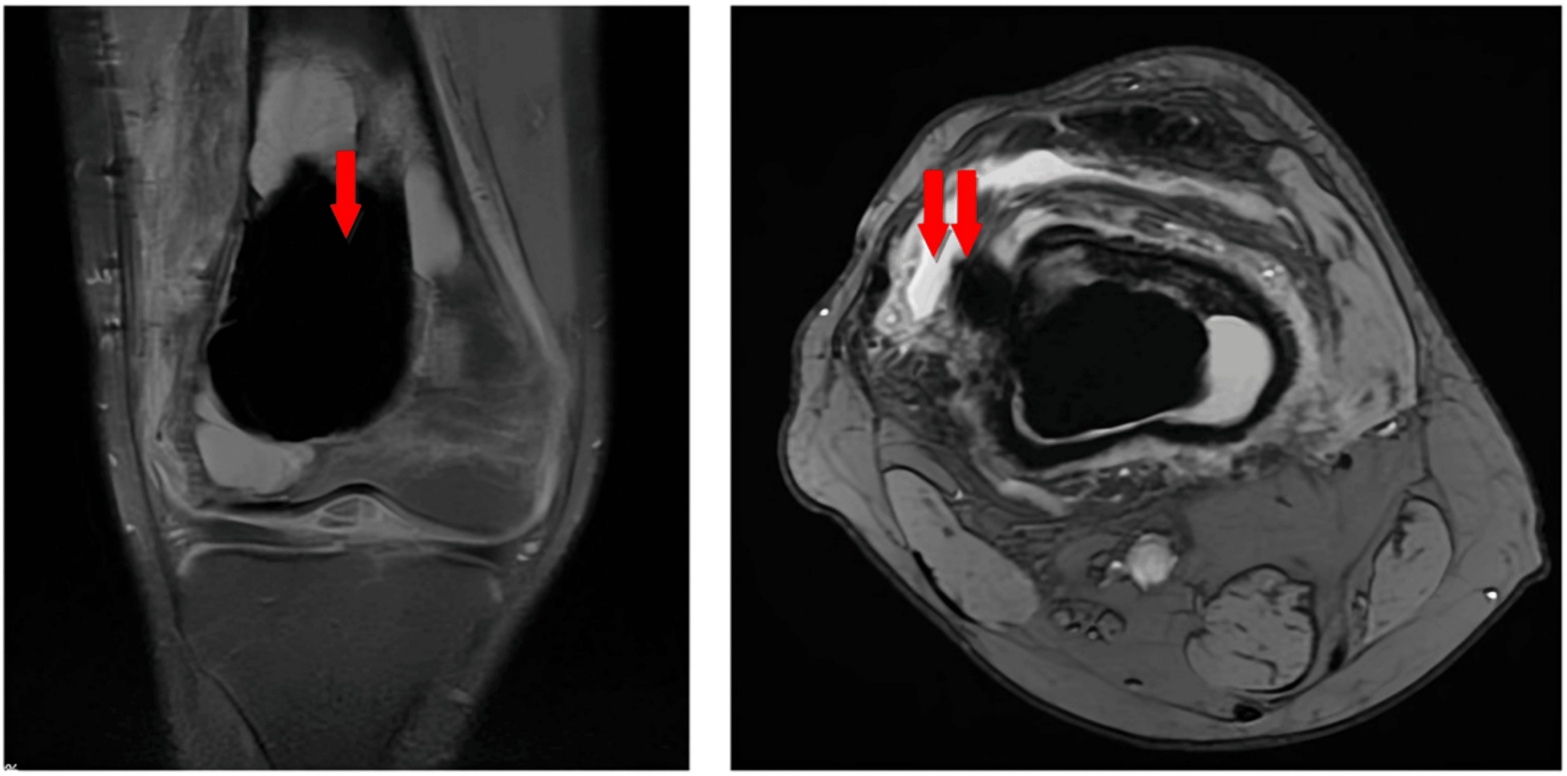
Preoperative MRI a. Sagittal section showing a lesion filled with fluid levels (arrow); b. Axial image showing a cystic-appearing lesion with mild surrounding soft tissue edema and irregular enhancement (arrows).
